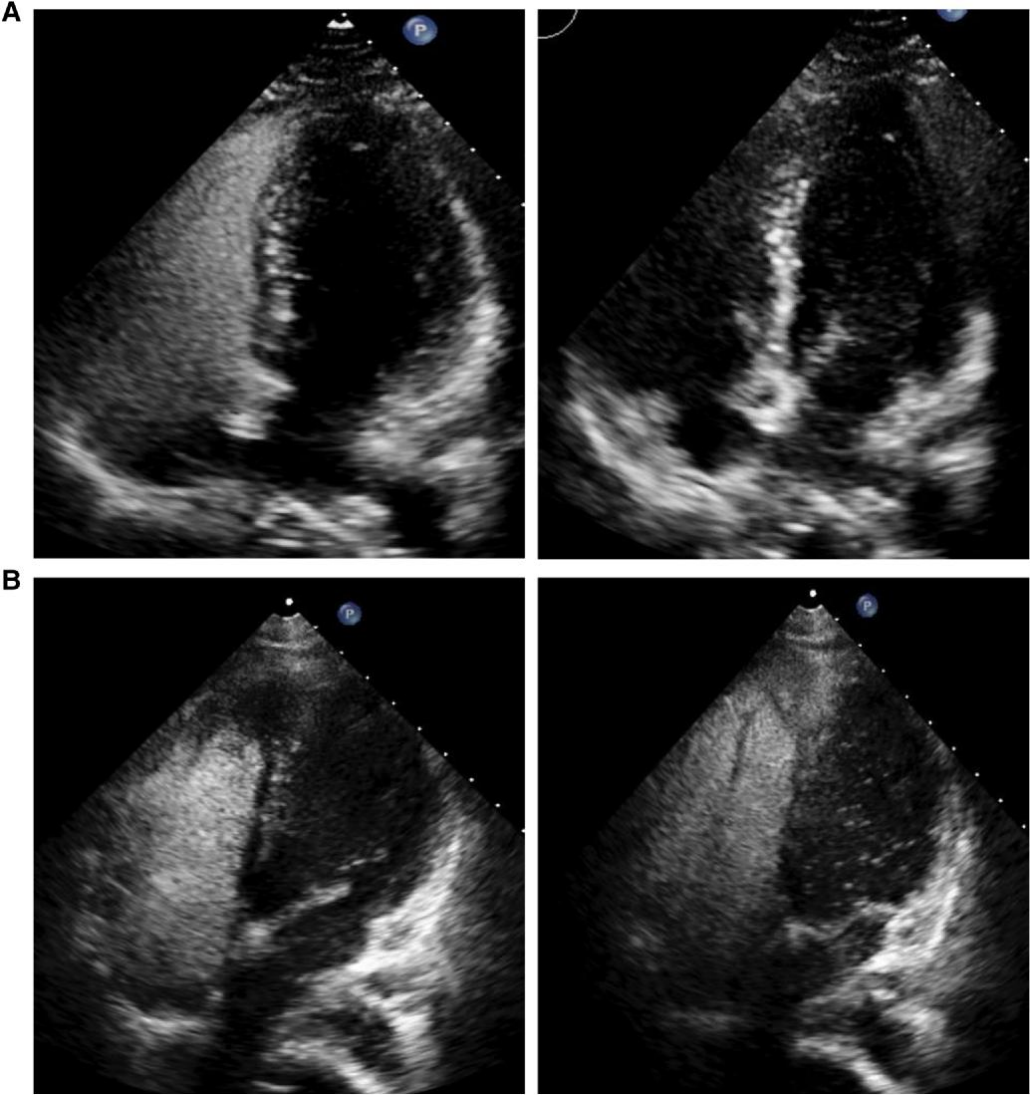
Contrast transthoracic echocardiography in Patient 1 (top left, top right images) and Patient 2 (bottom left, bottom right) showing late appearance of bubbles in left ventricle following peripheral vein injection.
